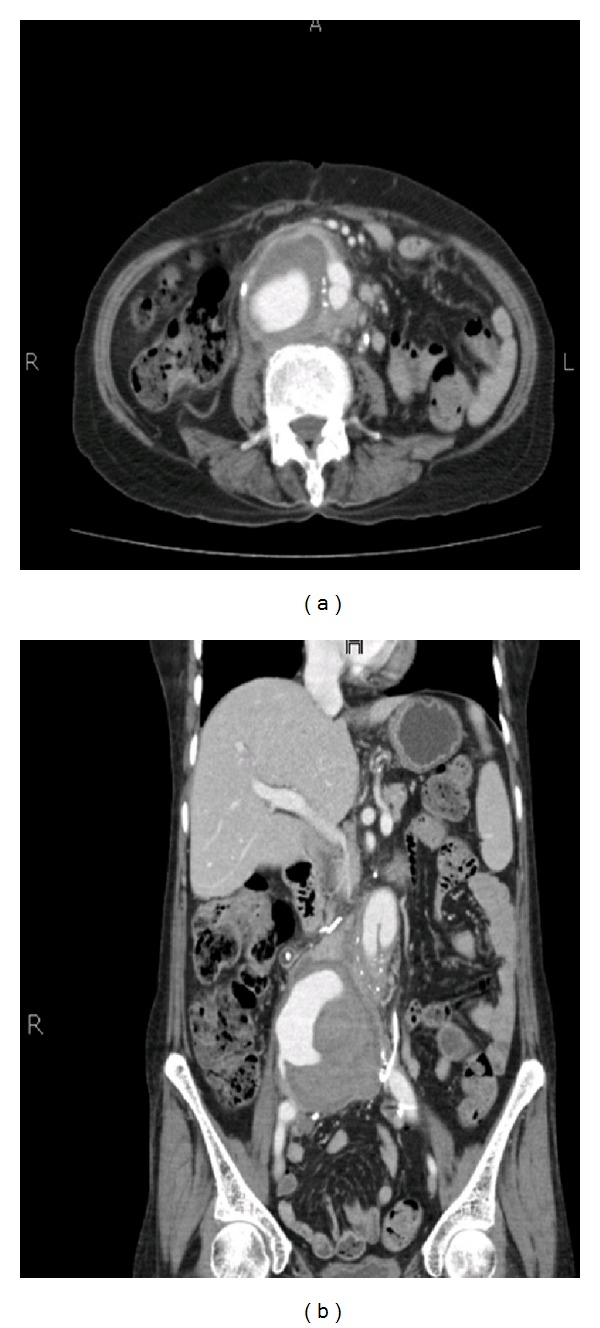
A 62-year-old woman with prior history of surgical repair of abdominal aortic aneurysm with a bifurcated graft presented with distal anastomotic pseudoaneurysm. Enhanced CT obtained 2 years after surgery reveals a pseudoaneurysm of 7.8 cm in diameter with pooling of IV contrast within it, close to the distal anastomosis.
